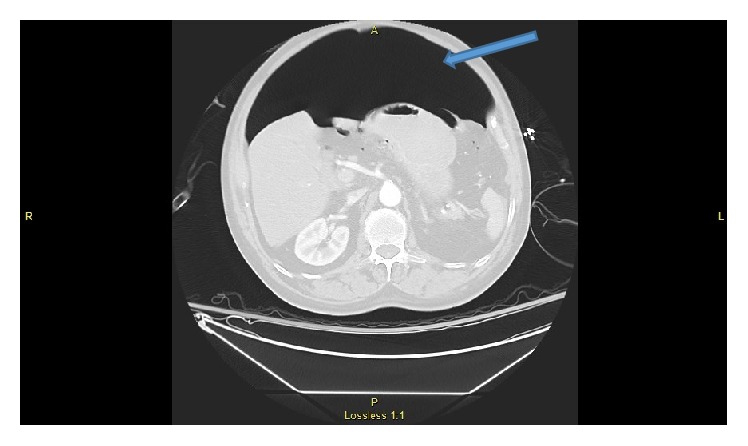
CT scan of abdomen showing large amount of air in the abdominal cavity.
